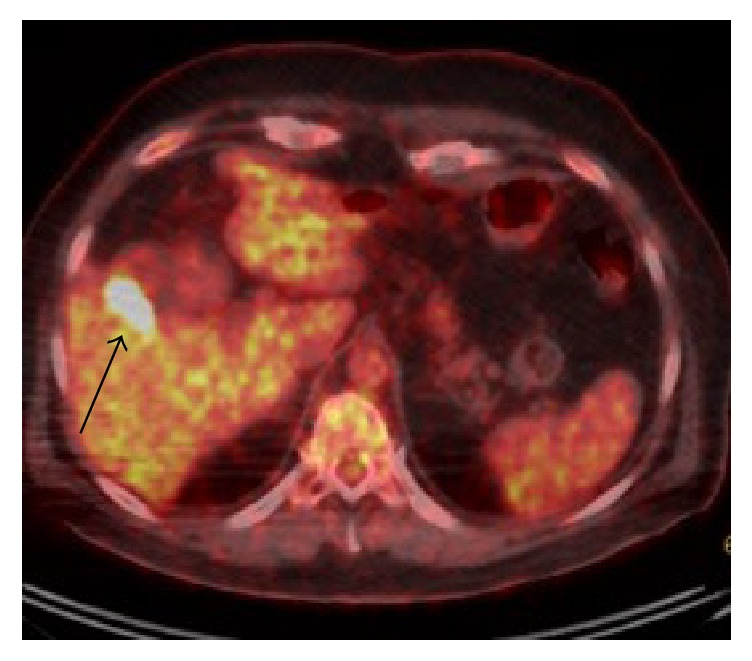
Fused PET/CT axial image demonstrating intense increased activity in the gallbladder (black arrow).
